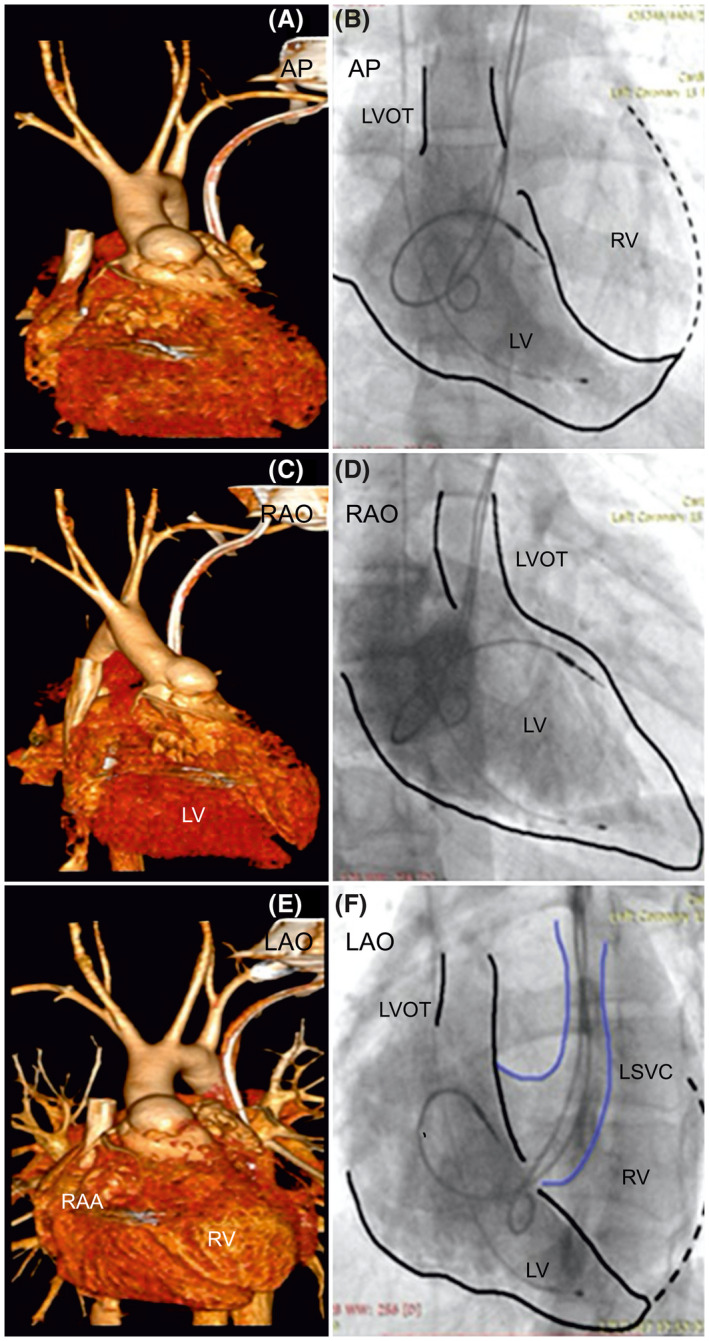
Three‐dimensional reconstruction of computed tomography and angiogram of the dual‐chamber pacing system in a patient with congenitally corrected transposition of the great arteries with persistent left superior vena cava. Computed tomographic three‐dimensional reconstructions of the heart in (A) AP, (C) RAO, and (E) LAO views showing pacing lead tip position. (B), (D), and (F) show procedural angiograms in equivalent views as the top panel. The ventricular lead is in the high‐septal location in the morphological left ventricle, and the atrial lead is in the right atrial appendage. AP, anteroposterior; LAO, left anterior oblique; LV, left ventricle; LVOT, left ventricular outflow tract; LSVC, left superior vena cava; RAA, right atrial appendage; RAO, right anterior oblique; RV, right ventricle
